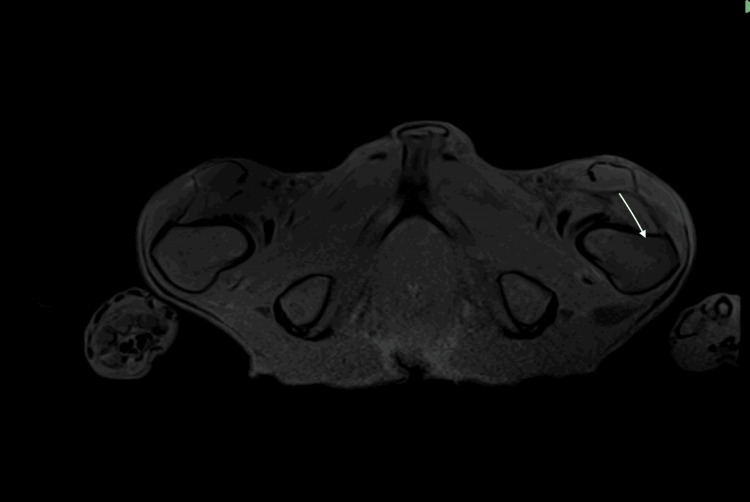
MRI of the Pelvis MRI of the pelvis demonstrating serous marrow atrophy, with diffuse loss of normal fatty marrow signal in the pelvic bones and proximal femora. The surrounding musculature appears preserved, with no fatty infiltration or inflammatory change.
